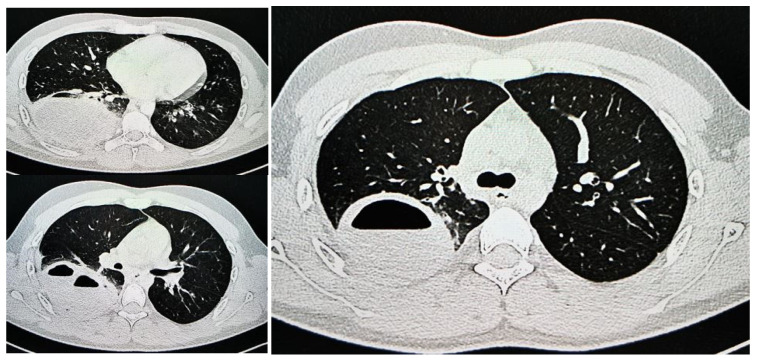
Seventeen-year-old male adolescent with pulmonary abscess: CT scan (transverse plane) with contrast performed, which describes oval mass in inferior right lobe, measuring circa 10 cm × 7 cm × 12 cm, with some round–oval parts containing air–fluid levels, circumscribed by walls and septa with varying thickness; pleural effusion of maximum thickness 10 mm.
